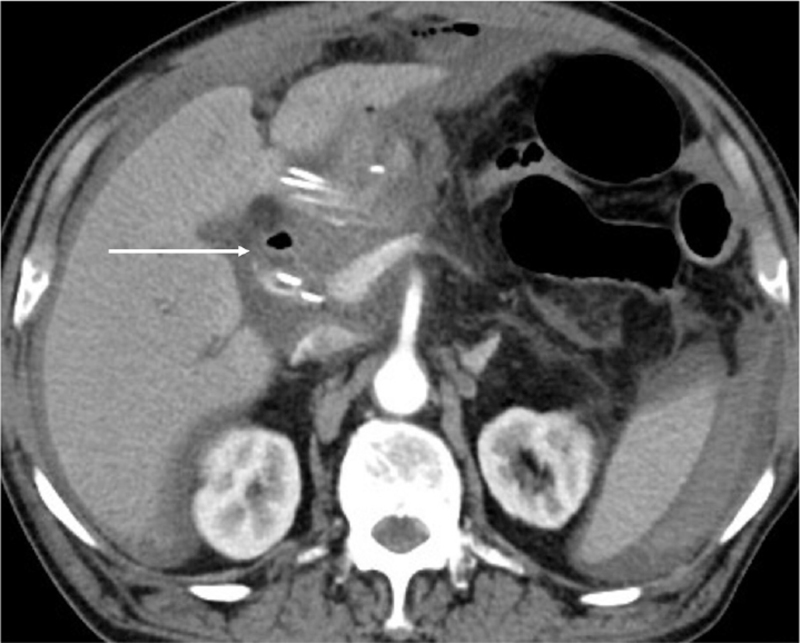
Arrow: CT angiography showed hemoperitoneum with no active extravasation of contrast. CT = computed tomography.
